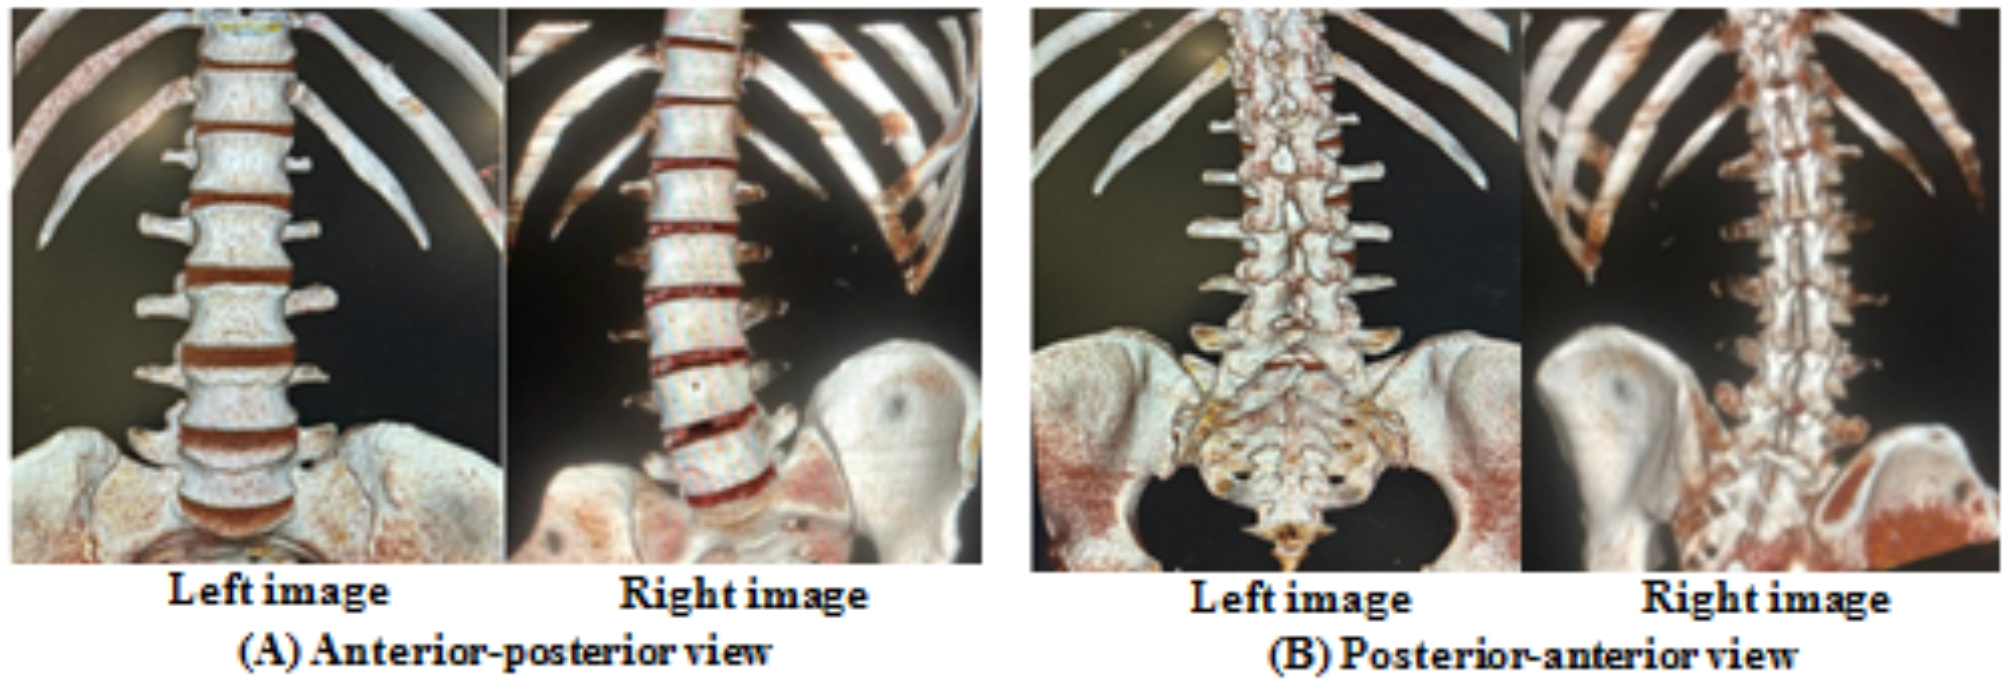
Lumbar 3D-CT scan images; right images from the modified reversed contralateral axial rotation (MRCAR) position and left images from the supine position
